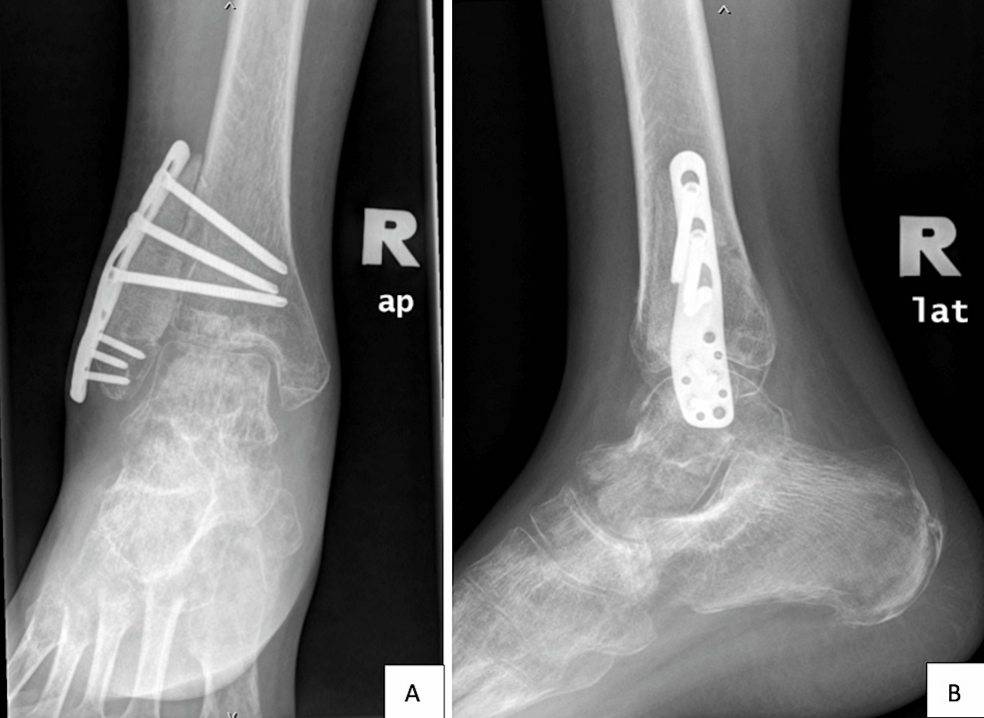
One-year post-operative radiograph showing that the autograft is not united to the tibia bone in the anterior-posterior (A) and lateral views (B)
